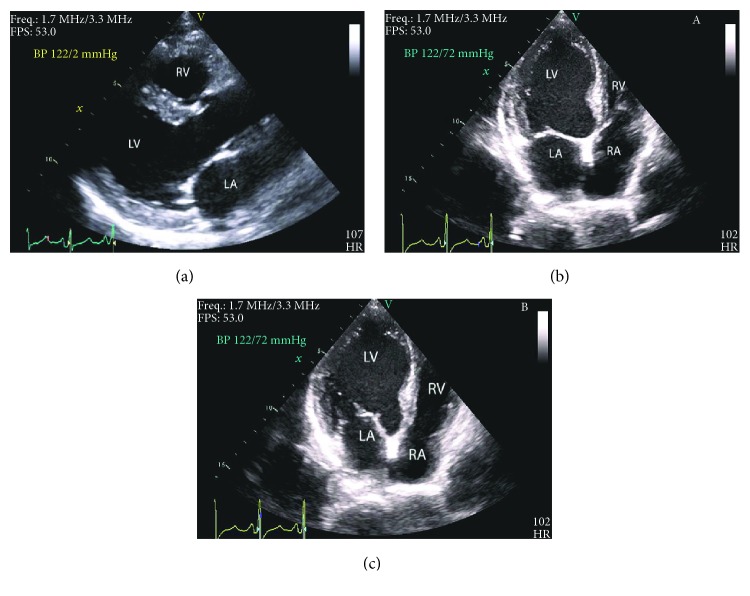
Transthoracic echocardiogram of the patient in parasternal long axis view (a) demonstrating an enlarged left ventricular cavity, in apical (four-chamber) view demonstrating a mildly abnormal end systolic (b) and normal end diastolic (c) left ventricular dimension (RV: right ventricle; LV: left ventricle; LA: left atrium; RA: right atrium).
